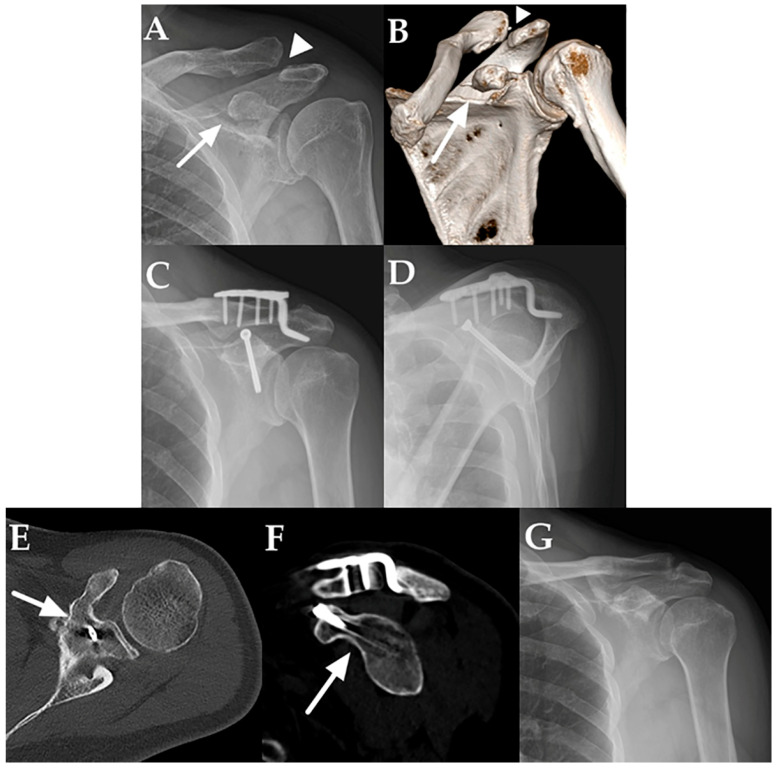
(A) Preoperative anteroposterior radiograph demonstrating a coracoid process (CP) fracture (arrow) with concomitant acromioclavicular (AC) joint dislocation (arrowhead). (B) Preoperative computed tomography (CT) with 3D reconstruction confirming the CP fracture (arrow) and associated AC joint dislocation (arrowhead). (C,D) Postoperative anteroposterior (C) and scapular Y view (D) radiographs demonstrating fixation of both lesions using a clavicular hook plate and a single 4.0 mm partially threaded cannulated screw. (E,F) CT images obtained 4.5 months postoperatively demonstrating complete union at the CP fracture site (arrow) on axial (E) and sagittal (F) views. (G) Follow-up radiograph after implant removal demonstrating no residual AC joint subluxation.
